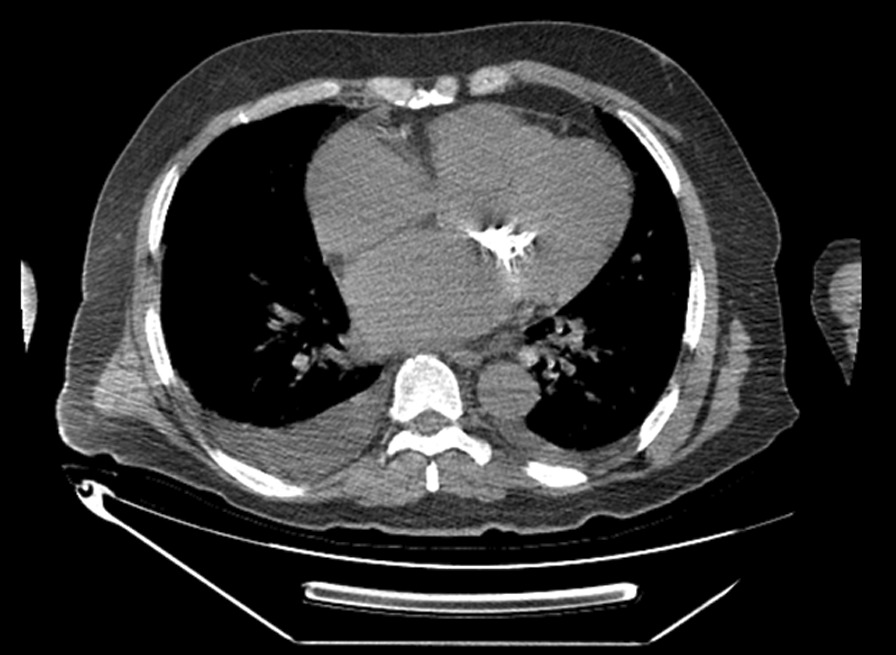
Chest computed tomography confirming mitral leaflet calcification
